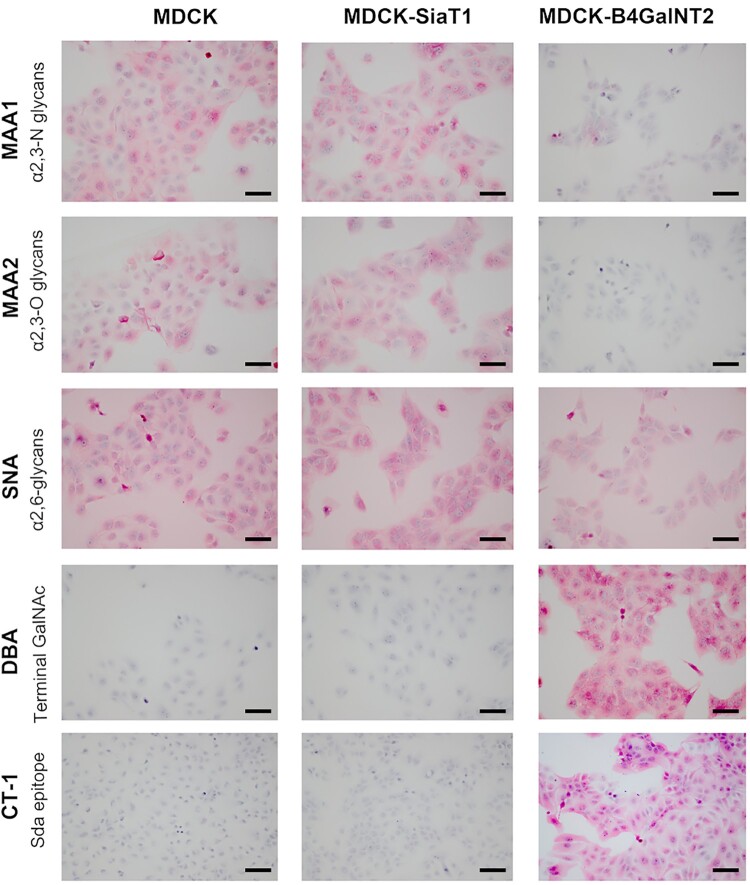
Immunocytochemistry of MDCK, MDCK-SiaT1 and MDCK-B4GalNT2 cells with lectins MAA1, MAA2,SNA, and DBA and monoclonal antibody CT-1. Scale bar = 100 μm.
